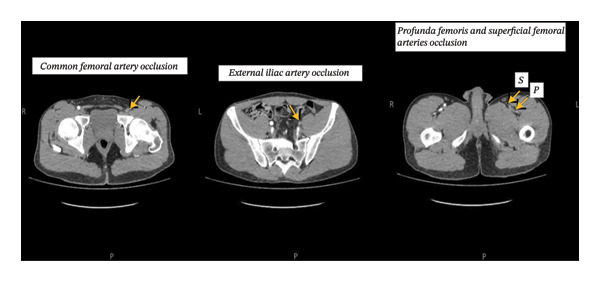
CT angiography of the left lower extremity showing complete occlusion of the common femoral artery, external iliac artery, profunda femoris, and superficial femoral arteries. No evidence of aneurysmal rupture or distal ischemia is noted, consistent with vascular Behçet’s disease.
